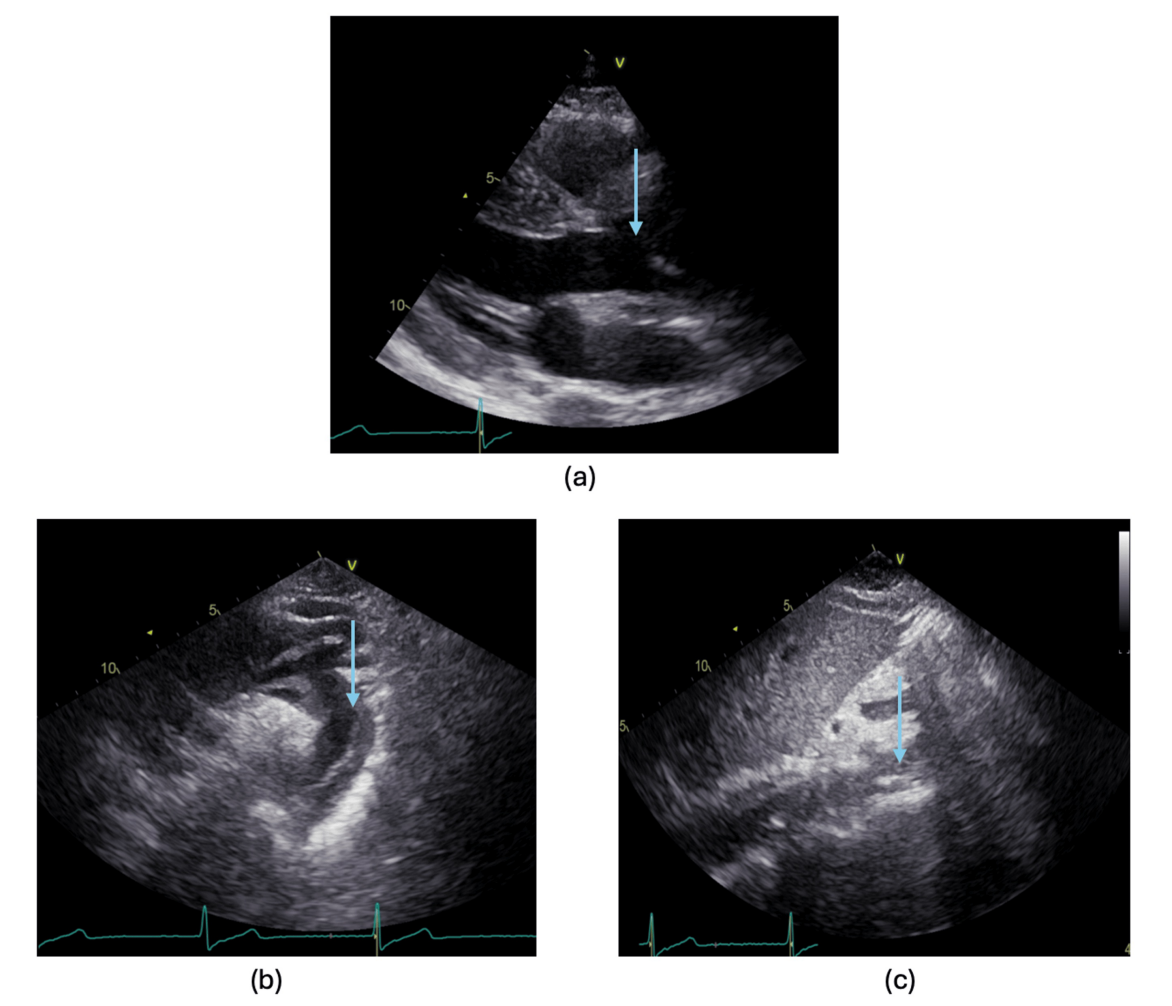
Echocardiograph images demonstrating aortic dissection (a) Parasternal long-axis echocardiographic view demonstrating a dissection flap at the aortic root level (blue arrow); (b) suprasternal echocardiographic view showing aortic dissection at the aortic arch level and descending aorta (blue arrow); (c) subcostal echocardiographic view demonstrating aortic dissection at the abdominal aortic level (blue arrow).
